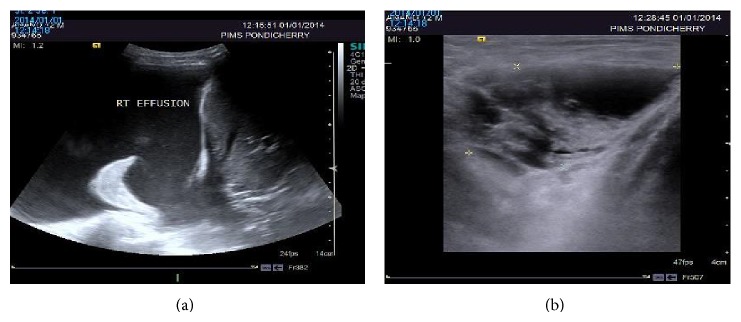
(a) Ultrasound examination of right lower chest showing right pleural effusion. (b) Ultrasound examination of soft tissue swelling in right axilla showing cystic mass with thick and thin septae due to lymphangioma.
